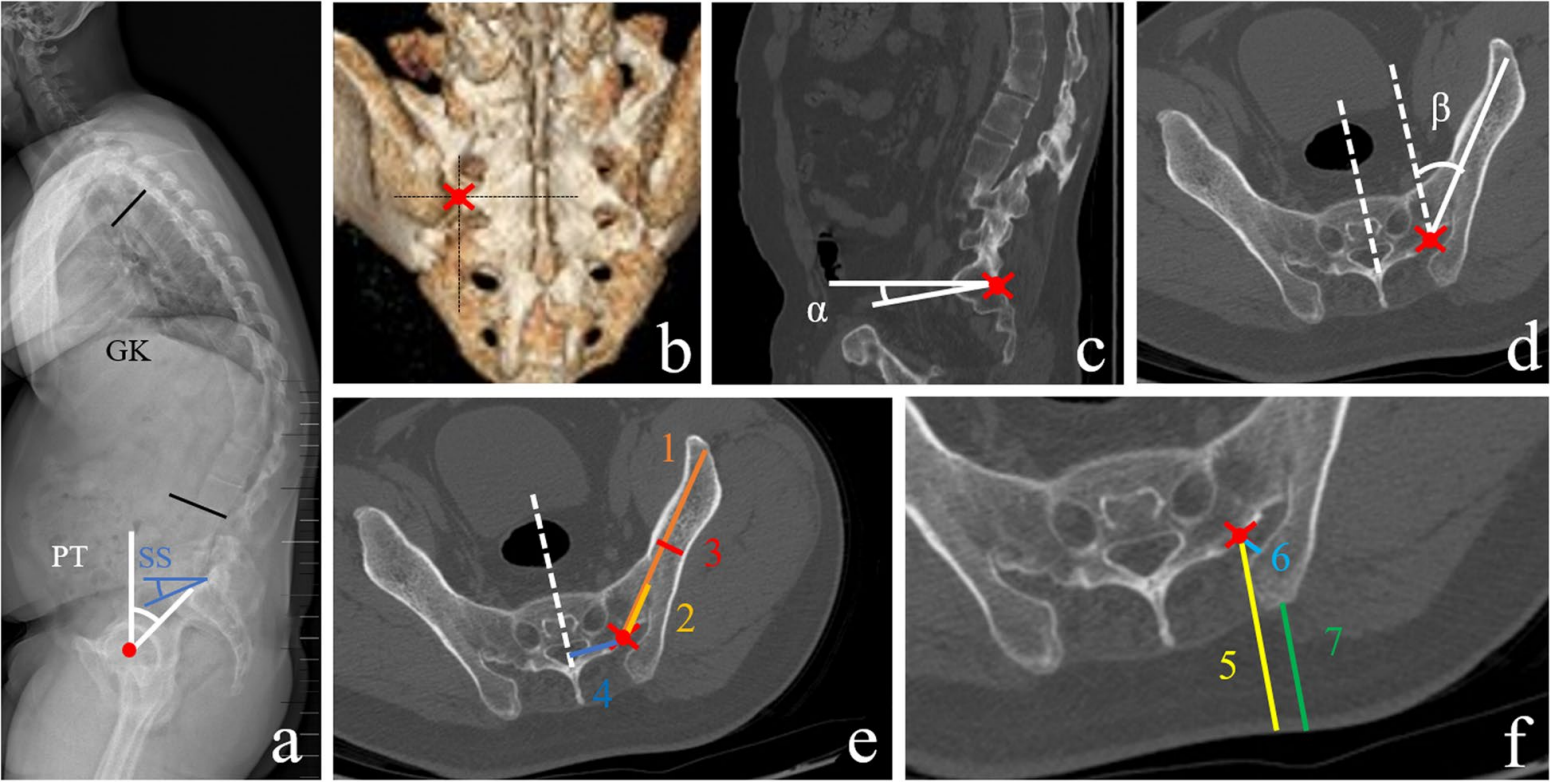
The measurements of sagittal parameters on lateral X-ray and S2AI pathway parameters on CT imaging for AS patients a GK, PT and SS were measured on a standard standing lateral radiograph. b Three-dimensional reconstruction of the pelvis. The starting point of the S2AI pathway was 1 cm inferior and 1 cm lateral to the S1 dorsal foramen. c CT imaging in the sagittal plane. Sag angle (angle α) is determined by the caudal trajectory angulation in the sagittal plane. d CT imaging in the transverse plane. Tsv angle (angle β) was defined as lateral trajectory angulation; e Max-length (line 1) determined by the maximal distance of the trajectory from S2 ala to the anterior inferior iliac spine; Sacral distance (line 2) was defined as intrasacral trajectory length; Iliac width (line 3) was defined as the narrowest iliac width measured between the inner cortices; S2 midline (line 4) was measured from the starting point lateral to the middle line of S2; f Skin distance (line 5) was measured from the starting point lateral to the skin; Iliac wing (line 6) was defined as the distance of the starting point lateral from the nearest iliac wing; PSIS distance (line 7) was measured from PSIS to nearest skin
